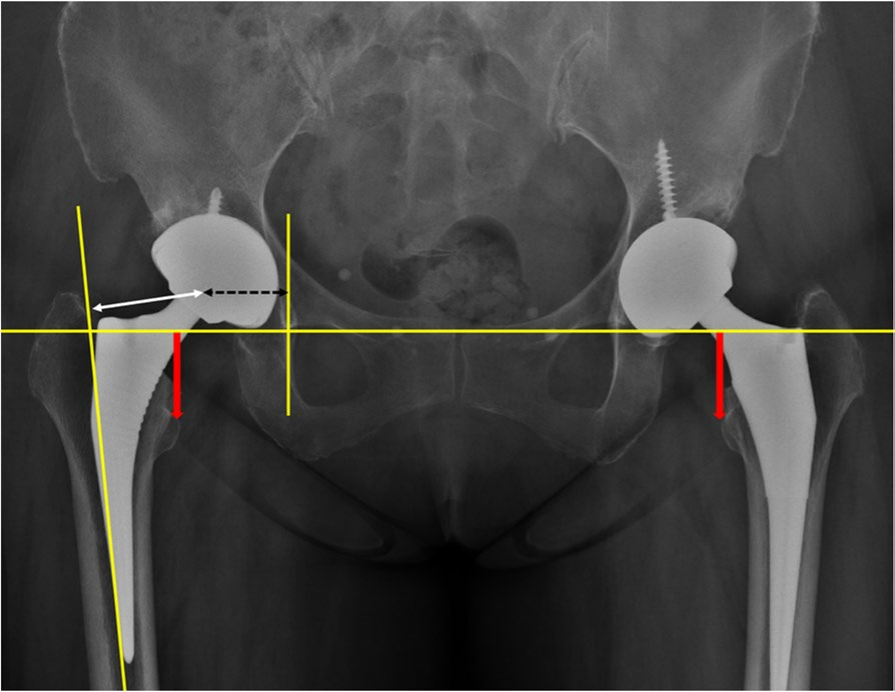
Radiographical measurement of LLD (thick red arrow), femoral offset (thin white arrow), acetabular offset (black dotted arrow), and combined offset (sum of femoral and acetabular offset)
